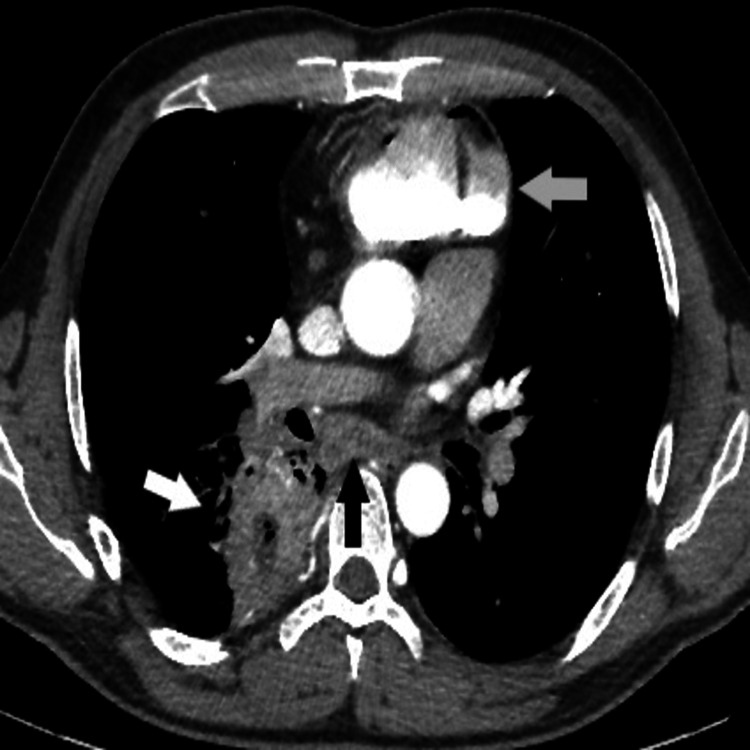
Computed tomography. White arrow- lung abscess, black arrow- oesophagus, grey arrow- contrasted retrosternal colon.
